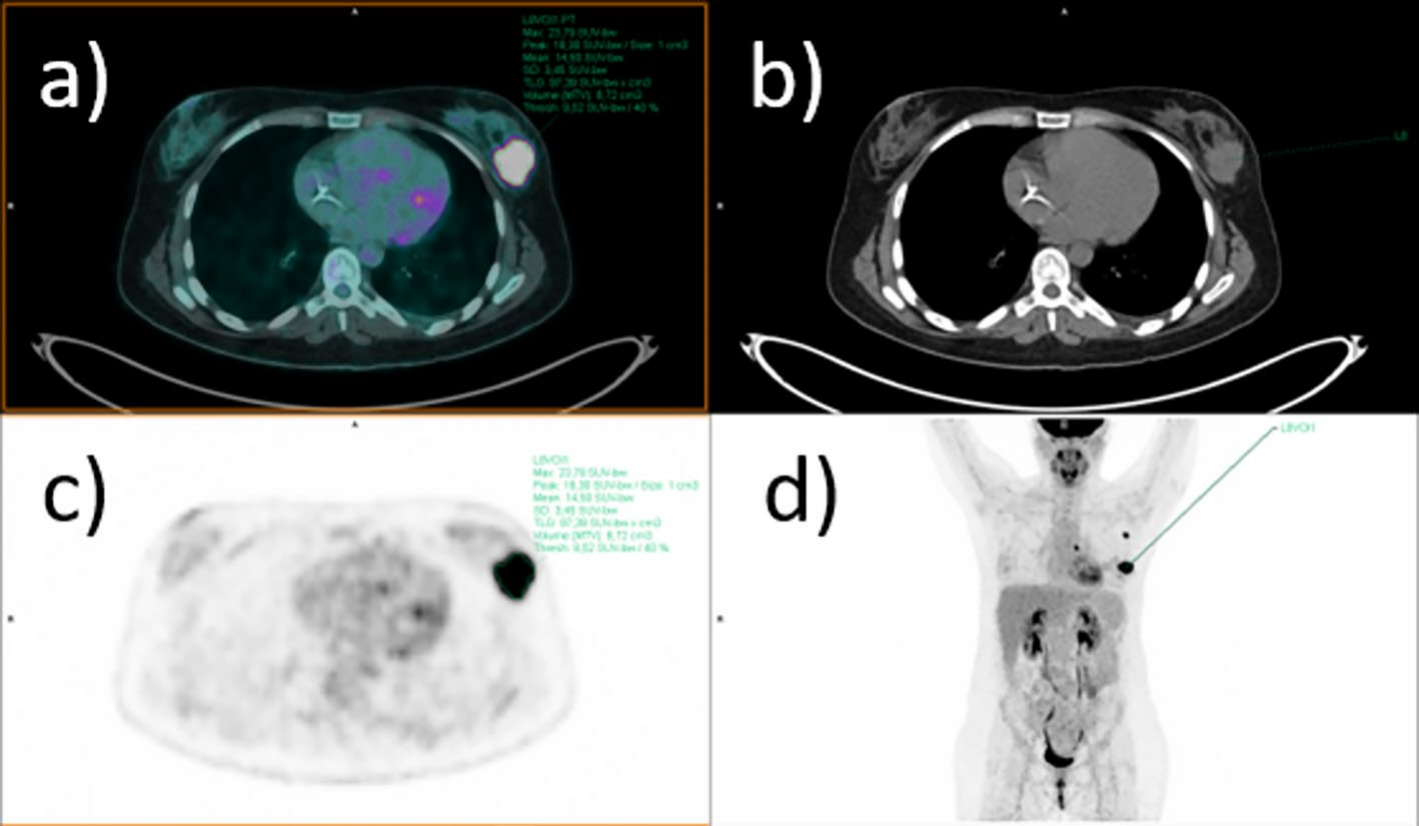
Baseline [^18^F]FDG PET/CT axial fused (a), CT (b), PET images (c) and MIP (maximum intensity projection) (d) of a 31 years-old women affected by TNBC. The exam shows focal [^18^F]FDG uptake (SUVmax = 23.8) in correspondence of the primary BC, as well as in a left axillary and in a homolateral internal mammary lymph nodes. The patient’s stage is therefore cT2;cN3. The patient achieved pCR after NAC
